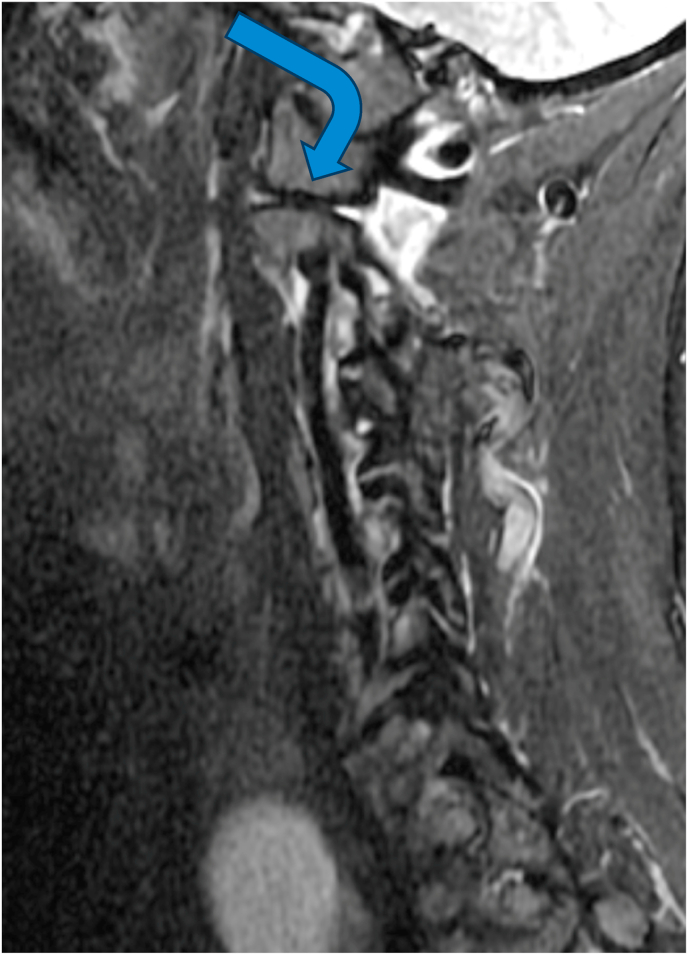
MRI showing no abnormalities in the left C1-2 joint (curved arrow).
